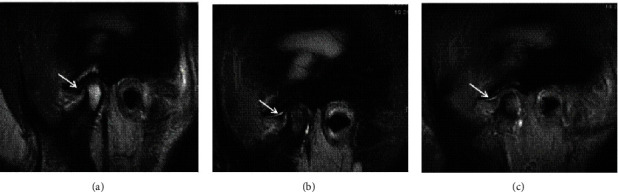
Sagittal MR images of the same patient using (a) FSE T2-W showing no effusion whereas (b) FS T2W and (c) 3D FIESTA-C show a moderate effusion in the superior joint compartment (arrows).
